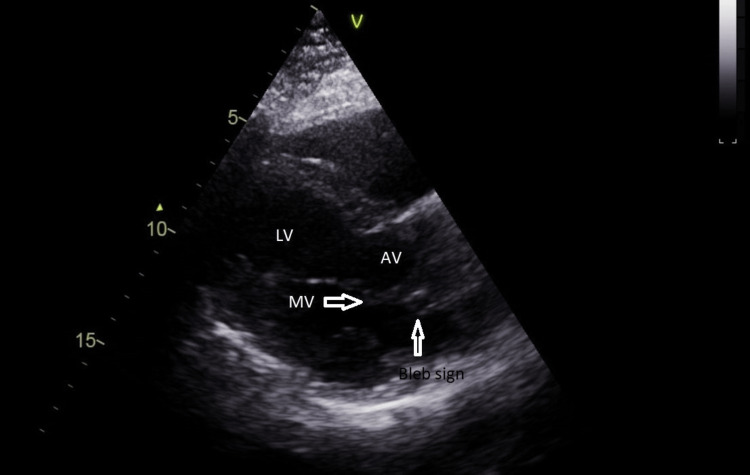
Conventional transthoracic parasternal long-axis view showing a small circle beneath the non-coronary cusp. Transthoracic bleb sign indicated by arrow. LV: Left ventricle; MV: Mitral valve; AV: Aortic valve.
